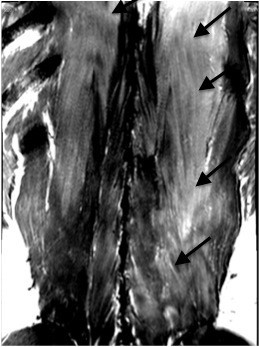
Magnetic resonance imaging (T1-weighted with gadolinium) showing edema and high signal intensity (arrows) in the region of the left multifidus and longissimus muscles.
